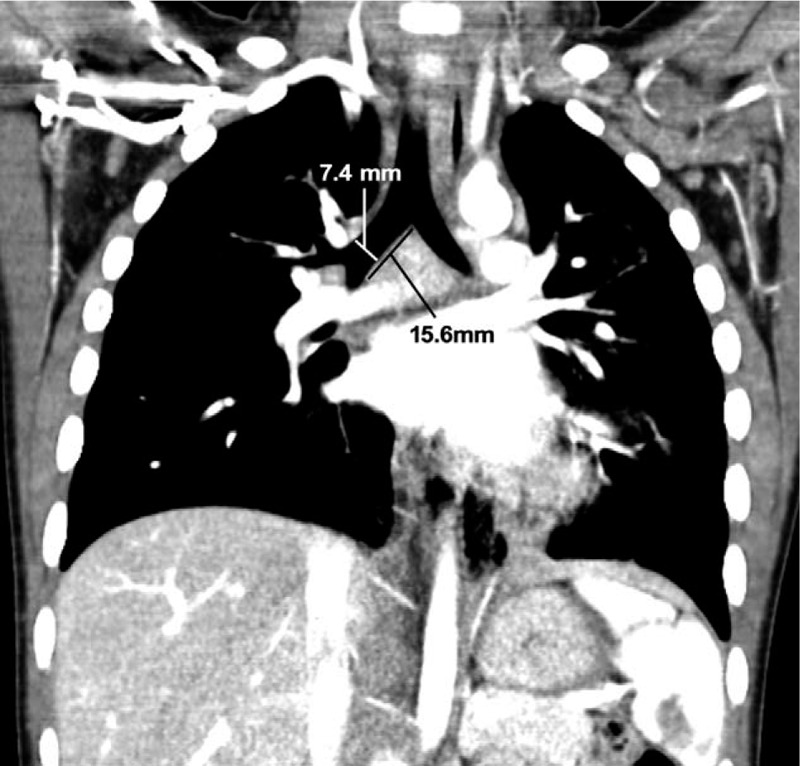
Contrast-enhanced chest computed tomography (CT). The largest diameter of right main bronchus is 7.4 mm. Length from carina to right upper lobar bronchus is 15.6 mm.
